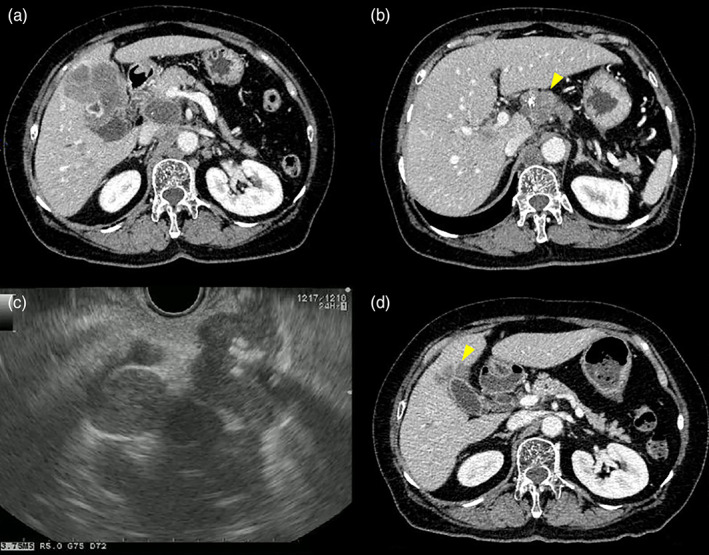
Contrast‐enhanced computed tomography (CECT) images showing (a) hypovascular gallbladder fundus tumor with a well‐defined margin accompanied by an intact overlying mucosa and liver invasion (67 mm), and (b) hilar lymph node (LN) enlargement with calcification (arrowhead). (c) Endoscopic ultrasound image from the stomach showing a hilar‐enlarged LN as a hypoechoic mass with calcification. (d) CECT images showing remarkable tumor shrinkage of liver invasion after six cycles of combination therapy with carboplatin and etoposide (arrowhead).
